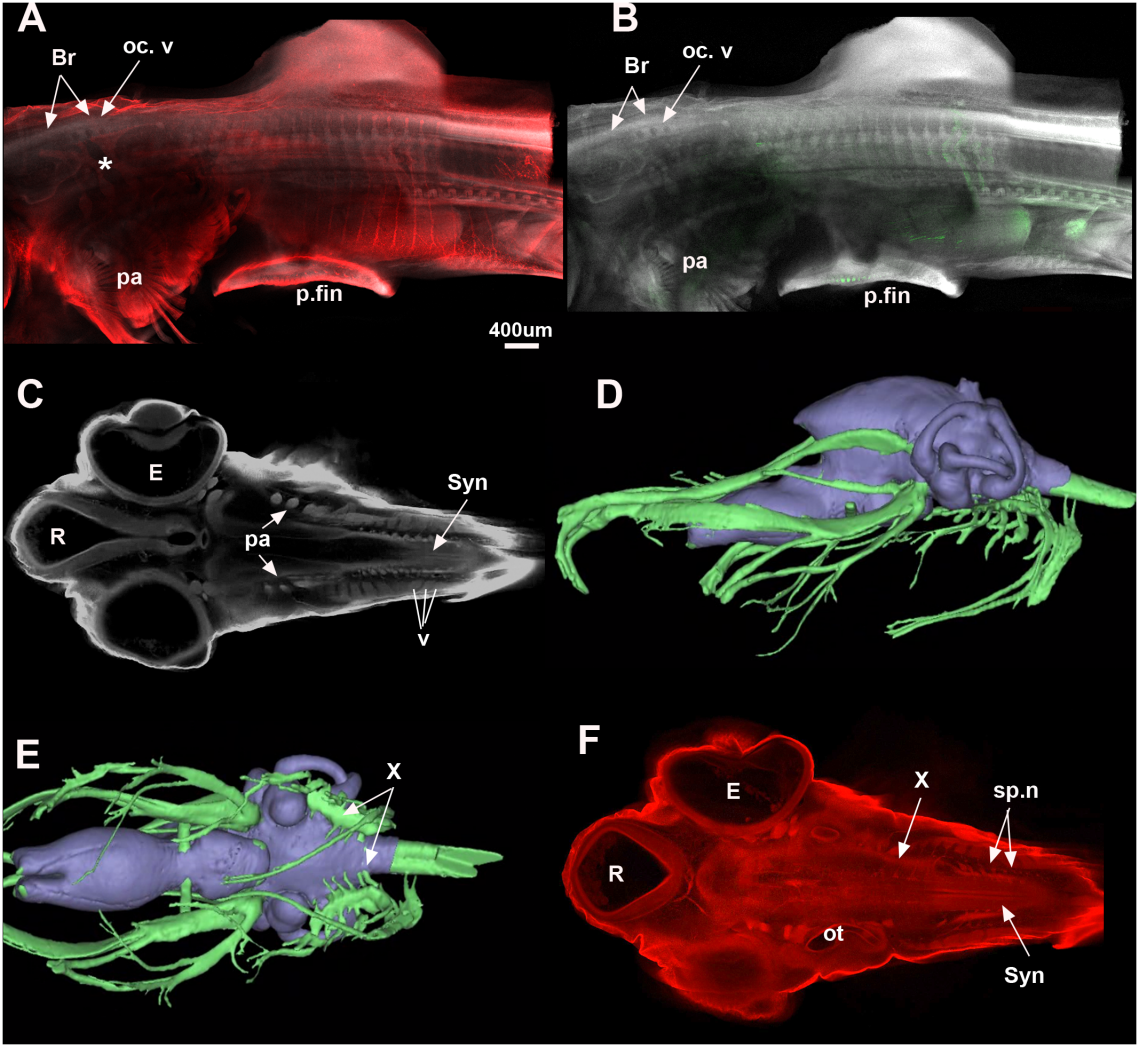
Callorhinchus milii*. A, B, Stage 29 (maximum projection, Sox9 red, DAPI white, Mf20 green) shows that the occipital vertebrae are still distinct from each other but that the braincase is now well developed. The posterior elements are still separate but show distinct dorsal and ventral elements for each vertebra. In stage 30 (C, F; one plane in Z, Sox9 red, DAPI white), the synarcual has started to form and can be recognised medially. The individual vertebral elements are still visible laterally (v). The vagus and accessory nerves are visible lateral to the mineralised braincase (C). D, E show distribution of the cranial nerves in a *C*. *milii* hatchling (Khonsari et al. 2013). Abbreviations as in previous figures, also Br, Braincase; E, Eye; R, Rostrum; Syn, synarcual. Images in Fig 6D and 6E taken as screenshots from a 3-D reconstructed CT scan model; Wikimedia Commons datafile, Khonsari et al. 2013. BMC Biology. doi:10.1186/1741-7007-11-27.
